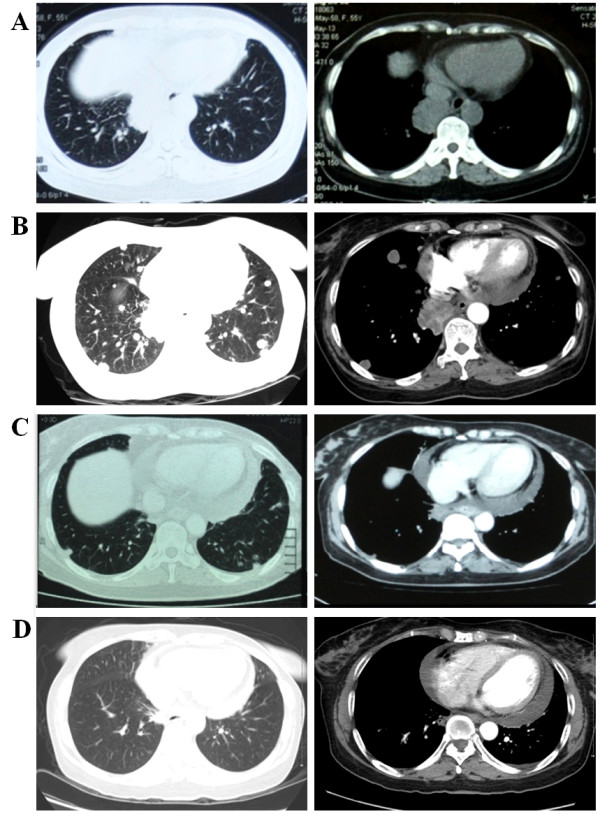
Chest computed tomography (CT) scans. Before the first chemotherapy treatment (May 2013) (A). After the second course of chemotherapy (B). After 26 days (C) and 11 weeks (D) of crizotinib treatment.
